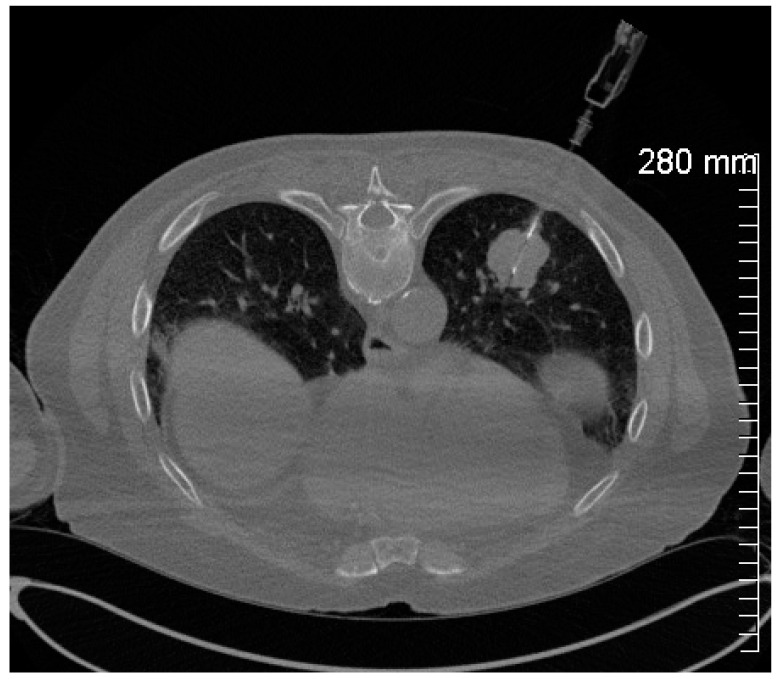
Intraprocedural CT fluoroscopic image demonstrating a 20-gauge core needle biopsy of the left lower lobe mass.
